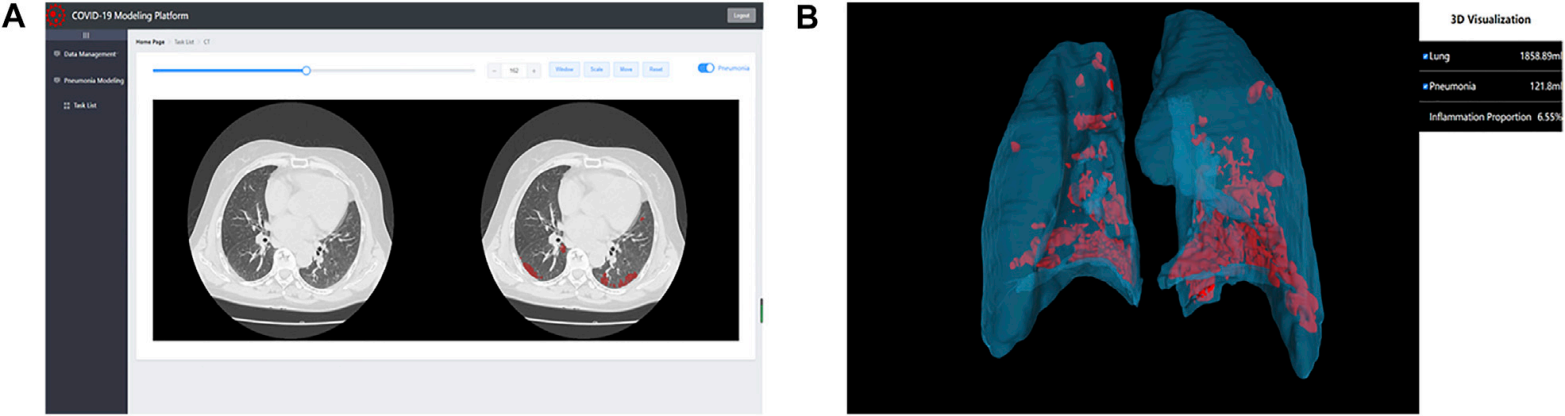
An online COVID-19 modeling platform for COVID-19 diagnosis. (A) shows the function of 2D CT reading. (B) shows the function of 3D model reading.
